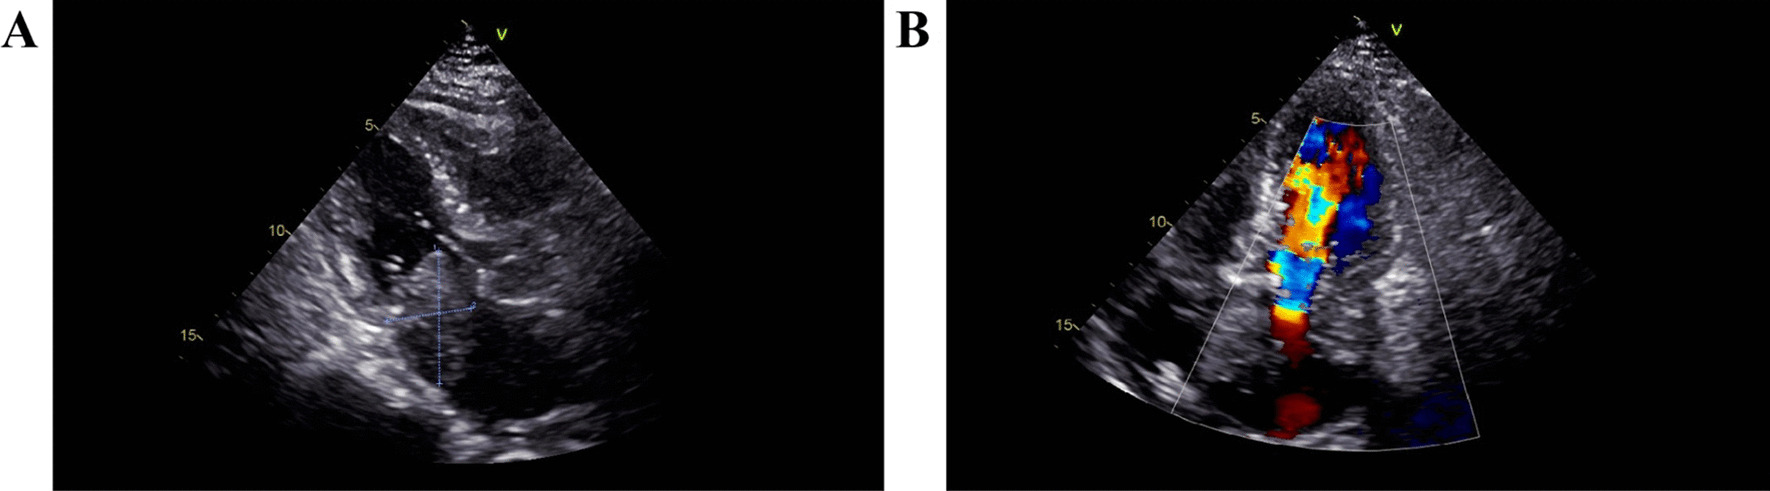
Preoperative ultrasound image of myxofibrosarcoma in the left atrium. (A) Two-dimensional ultrasound image of myxofibrosarcoma. (B) Ultrasound image of diastolic mitral flow in myxofibrosarcoma
